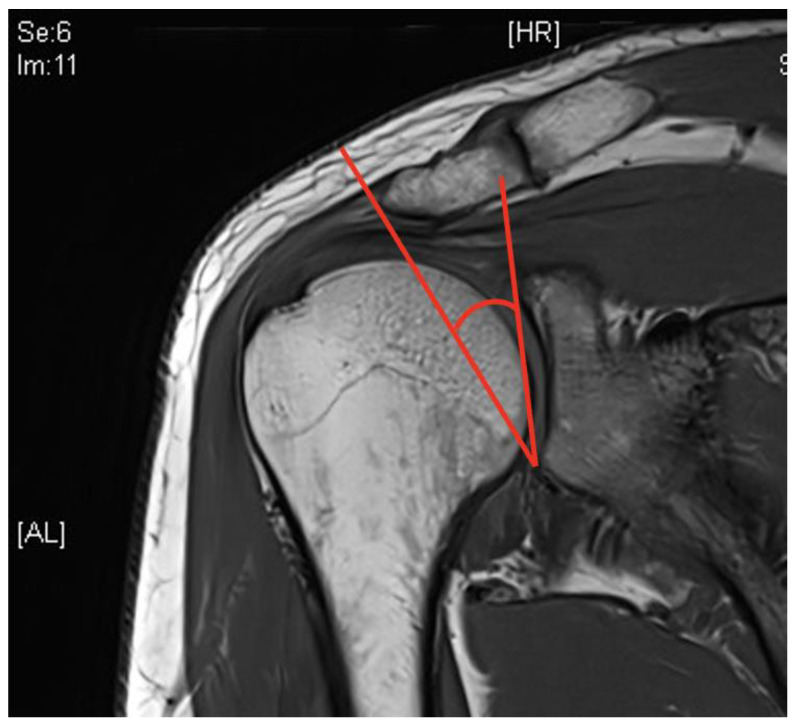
CSA depicted in an MRI in coronary sections (T1, 3 Tesla).
